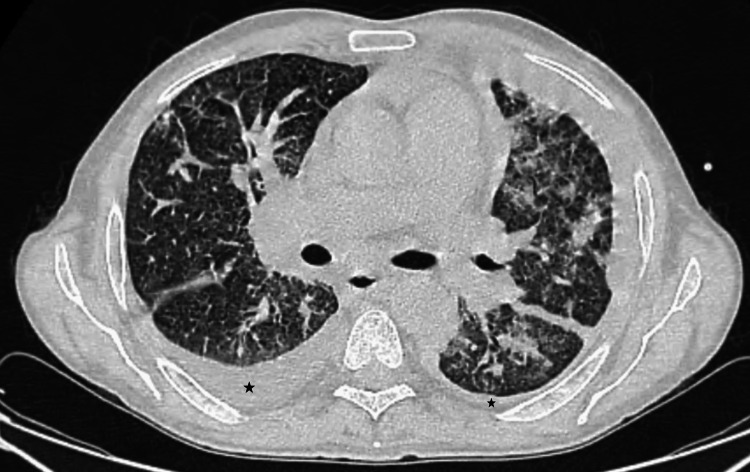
Case 2: Axial slice of a chest CT scan demonstrating bilateral interstitial involvement with septal thickening, micronodules of mixed distribution, and bilateral pleural effusion (stars).
